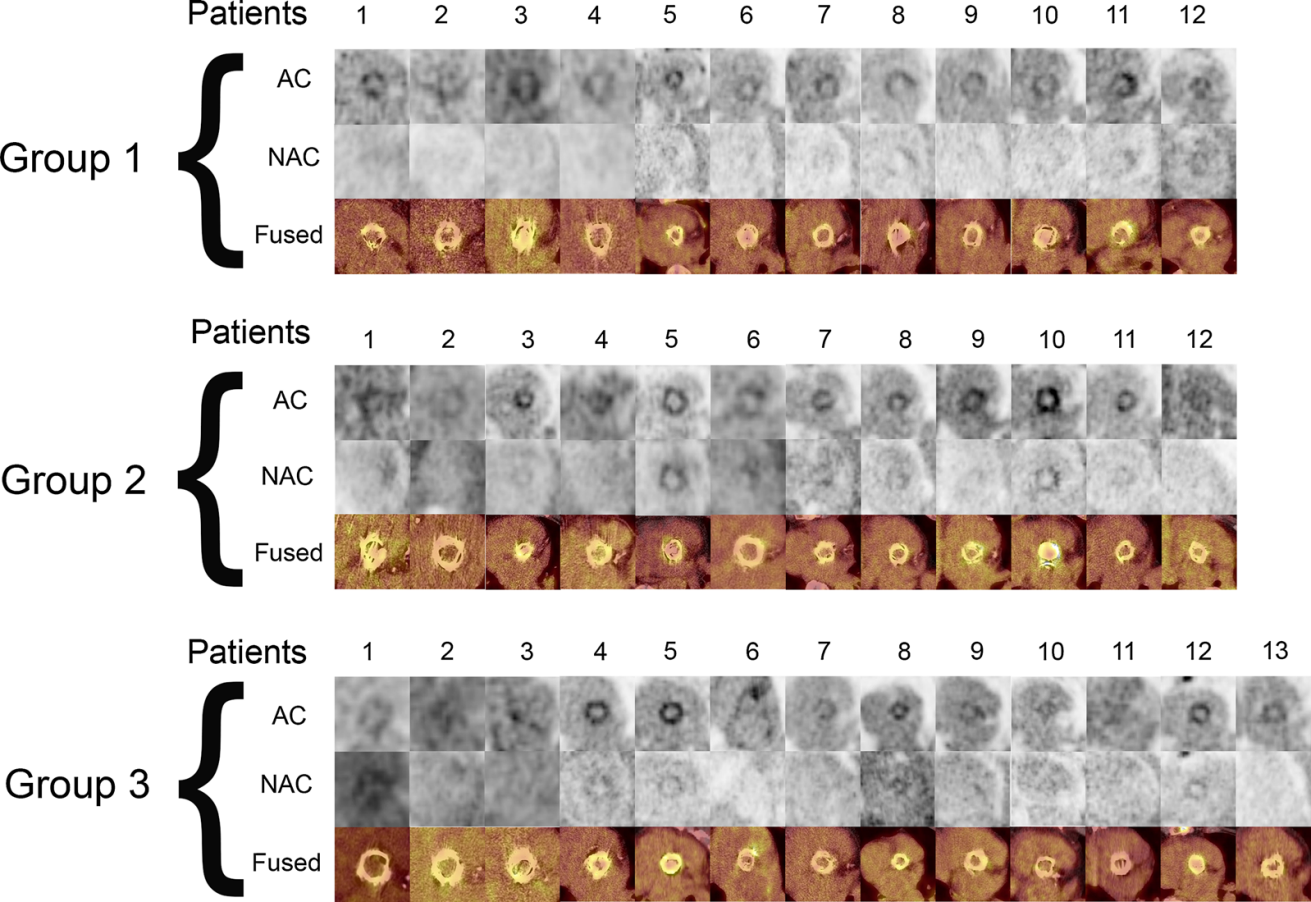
^18^F-FDG uptake around the PHV on reconstructed views in plane with the PHV of attenuation-corrected (AC) images, non-attenuation-corrected (NAC) and fused attenuation-corrected images with CT in all patients. Scaling was set the same for all AC images (0-7MBq)
